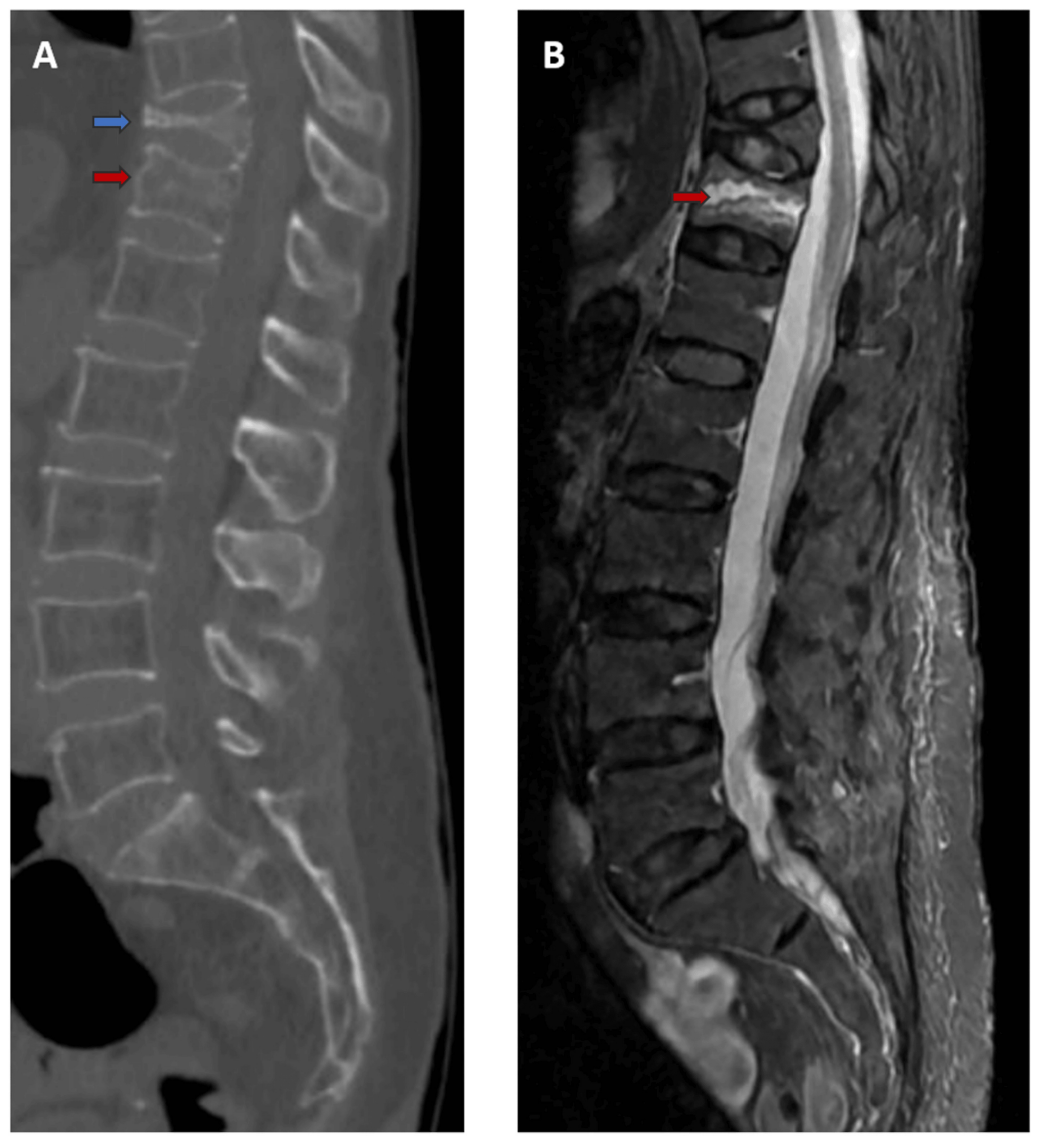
Vertebral compression fractures of the 11th (chronic) and 12th (acute) thoracic vertebrae Image A is a sagittal CT image showing compression fractures of the 11th (blue arrow) and 12th (red arrow) thoracic vertebrae. Image B is a STIR sequence MRI showing the same fractures and demonstrating the marrow edema (hyperintensity signal) of the 12th vertebral body (red arrow), which is indicative of acute injury. CT: computed tomography; MRI: magnetic resonance imaging; STIR: short tau inversion recovery
